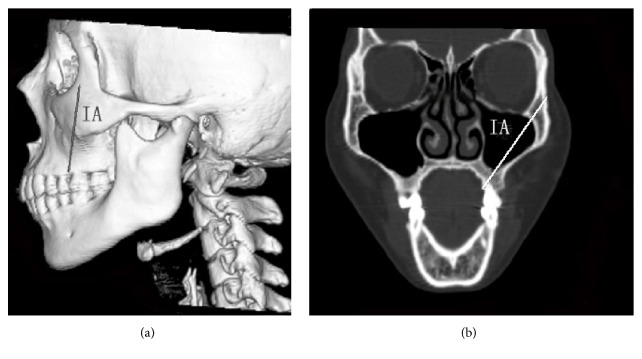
The implant axis (IA) on a 3D-CT image. (a) 3D-CT reconstruction image of the head; (b) CT image of the plane including IA.
